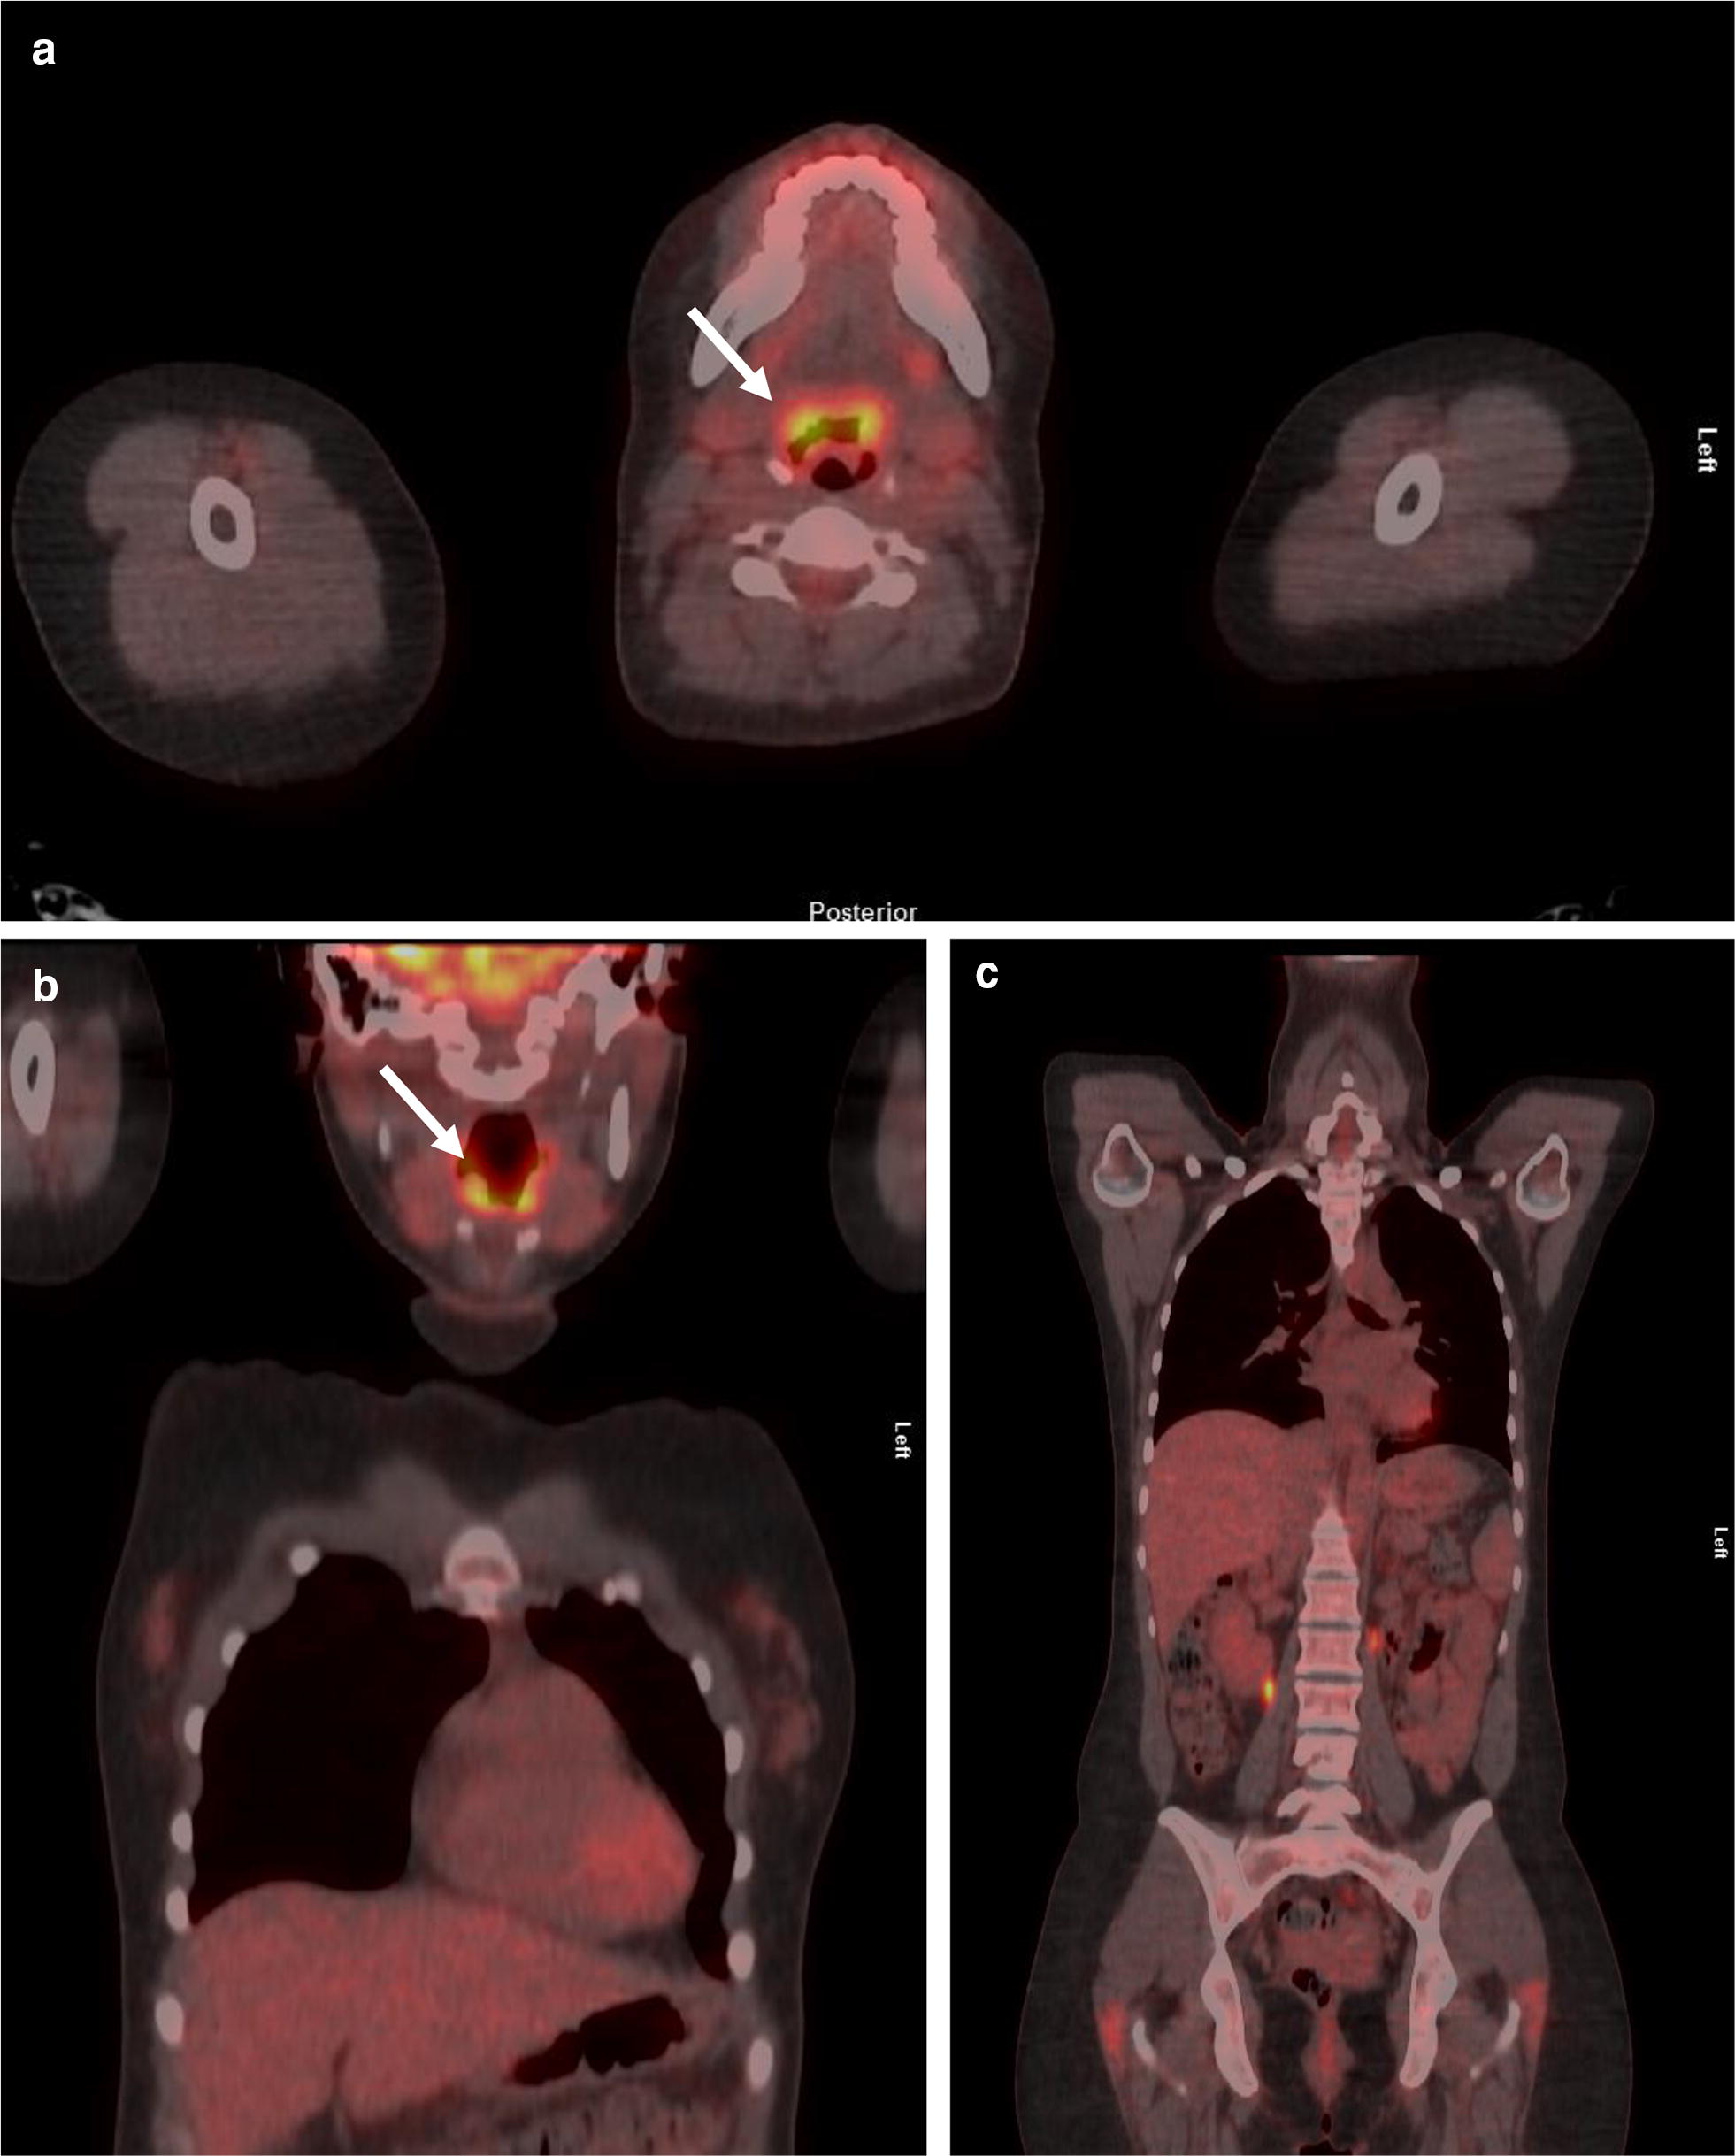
Positron emission tomography—computed tomography showed increased activity (arrows) in the lingual tonsil (a, b) without evidence of widespread sarcoidosis (c)
